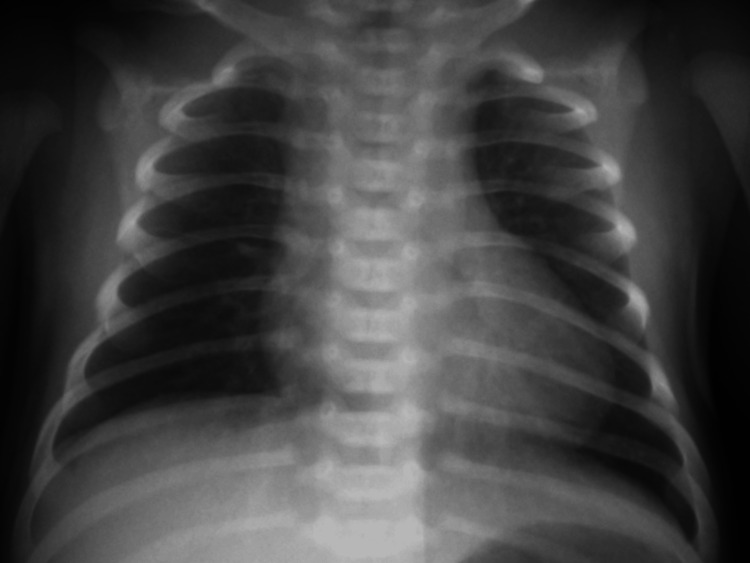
Chest radiography Chest radiography showed no abnormalities.
